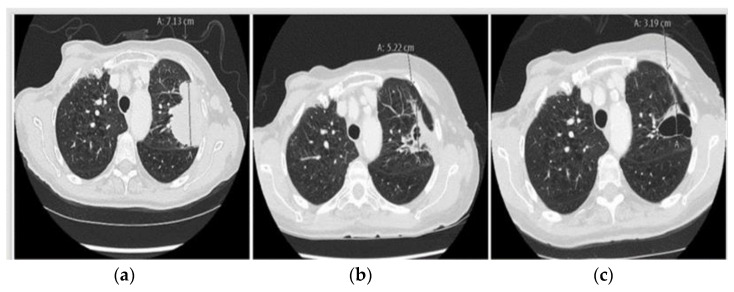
The figure presents three CT scans of the thorax under the treatment course of case 3. “A” represents the lung tumor. (a) Tumor progression on Osimertinib–Alectinib. (b) Significant tumor regression after three months of combination treatment with Crizotinib and Osimertinib. (c) Further tumor regression after six months of treatment with Crizotinib and Osimertinib.
